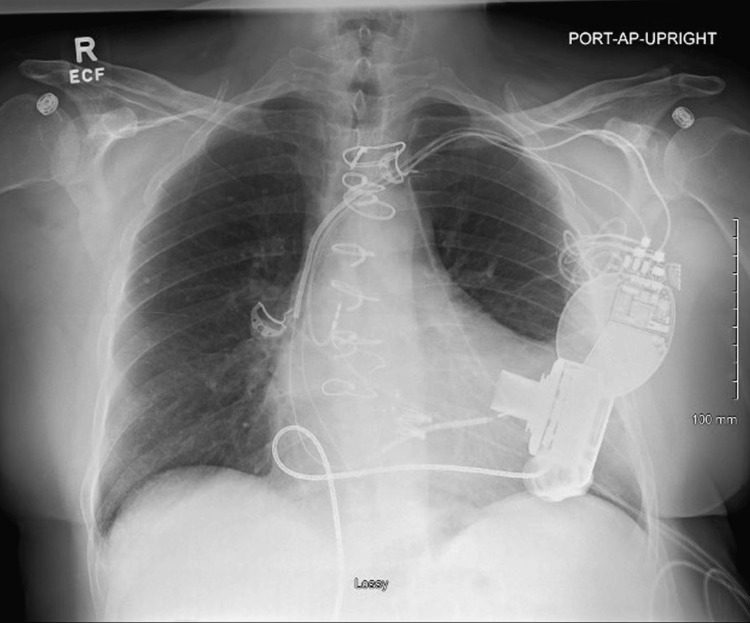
The portable AP X-ray view shows the left ventricular assist device in a stable position. Additionally, the left subclavian pacemaker and ICD are both confirmed to be in stable positions. AP: anteroposterior; ICD: implantable cardioverter defibrillator
